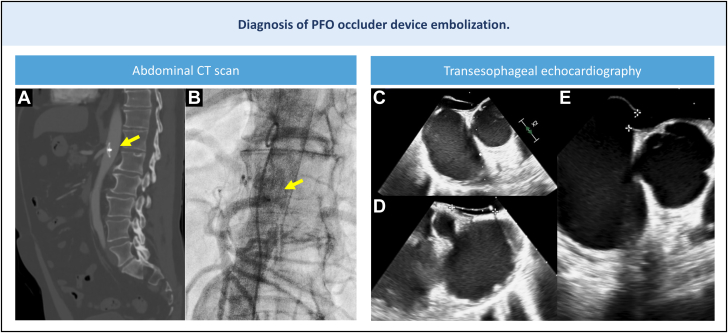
Non-invasive Imaging Demonstrating PFO Occluder Embolization (A and B) PFO device embolized into the abdominal aorta, depicted on CT scan and angiography (arrows). (C to E) TEE showing a large PFO. CT = computed tomography; PFO = patent foramen ovale; TEE = transesophageal echocardiography.
